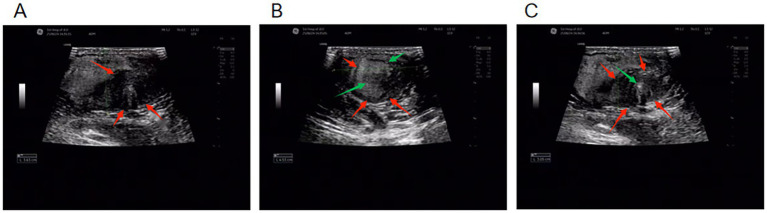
Gynecological color Doppler ultrasound examination image of the patient before admission: (A) Red arrow: boundary of the vulvar mass; (B) green arrow: slightly strong echo mass inside; (C) uneven echo of the mass.
